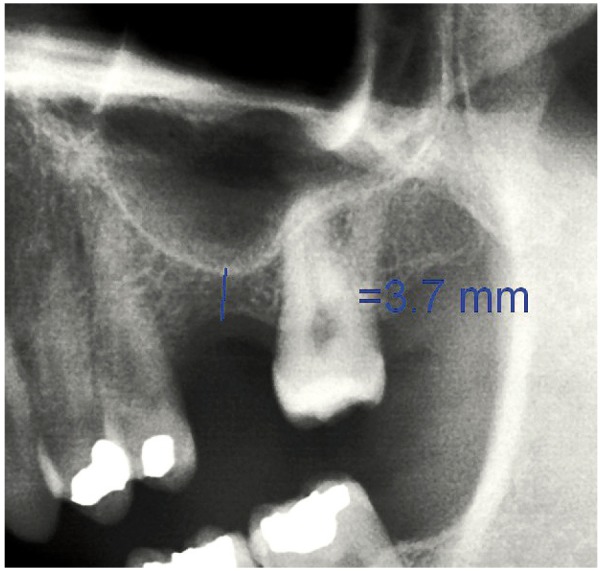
Case 1. 6. Preoperative panoramic X-ray view.
